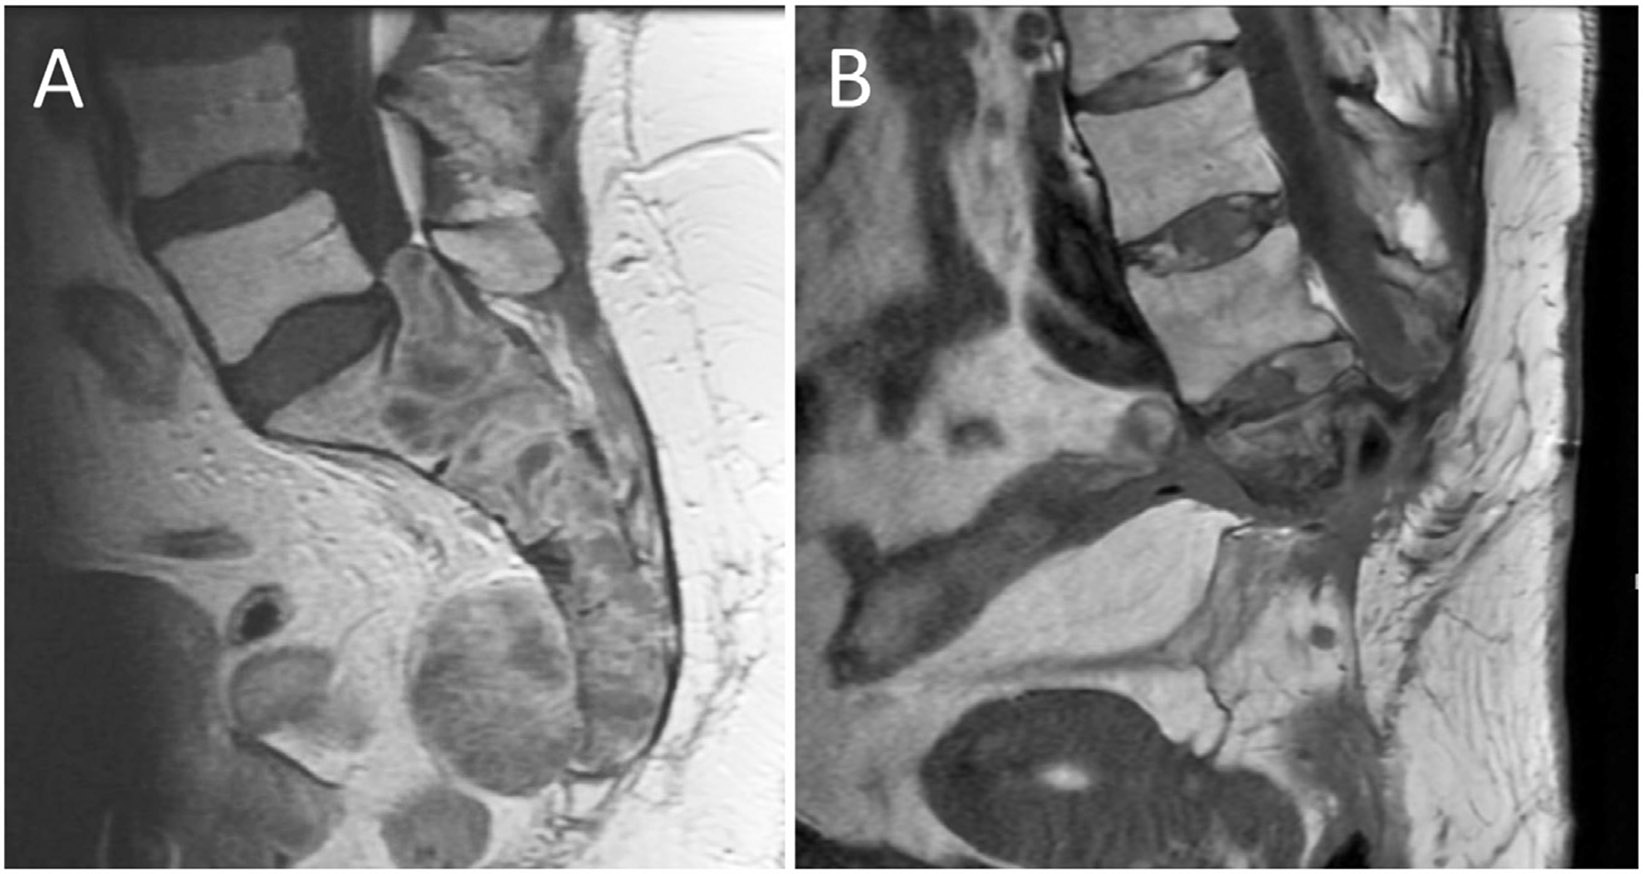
A representative case depicting (A) preoperative and (B) postoperative T1-weighted sagittal magnetic resonance imaging scans of a 49-year-old woman with a high sacral chordoma who underwent neoadjuvant 24-Gy single-fraction stereotactic body radiation therapy and surgical resection with negative margins. She is currently free of disease at 10 years.
